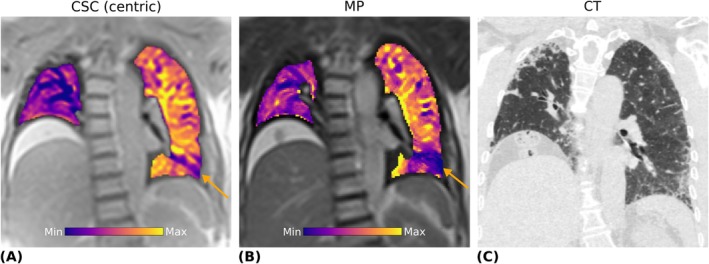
75‐year‐old female patient diagnosed with fibrosing hypersensitivity pneumonitis and elevated right hemidiaphragm. Ventilation maps indicate a strong functional impairment in the right lung, as well as hypoventilated regions affected by fibrosis in the lower lobe of the left lung (orange arrow). The ventilation maps were derived from the MP sequence (A) and the proposed CSC centric acquisition (B) with 10 mm slice thickness. The corresponding CT image demonstrates subpleural fibrosis in the left lower lobe (C).
